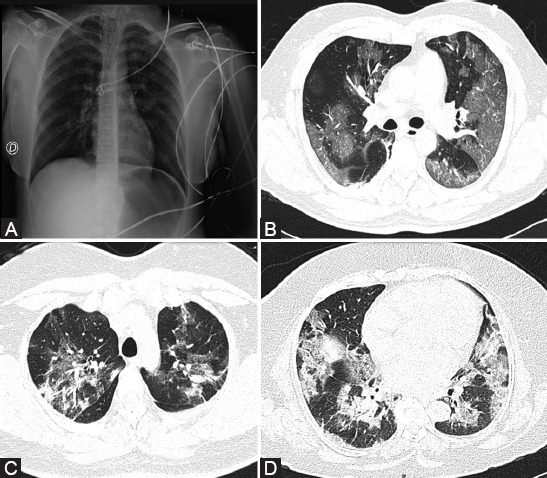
Classical images of X-Ray and CT-Scan from a female COVID-19 patient. A) Portable X-Ray with diffuse bilateral ground-glass patches. B) Chest CT-Scan with subpleural predominance ground glass sample (initial phase of the disease). C and D) Chest CT-Scan is showing a crazy paving and consolidation pattern (disease progression).
